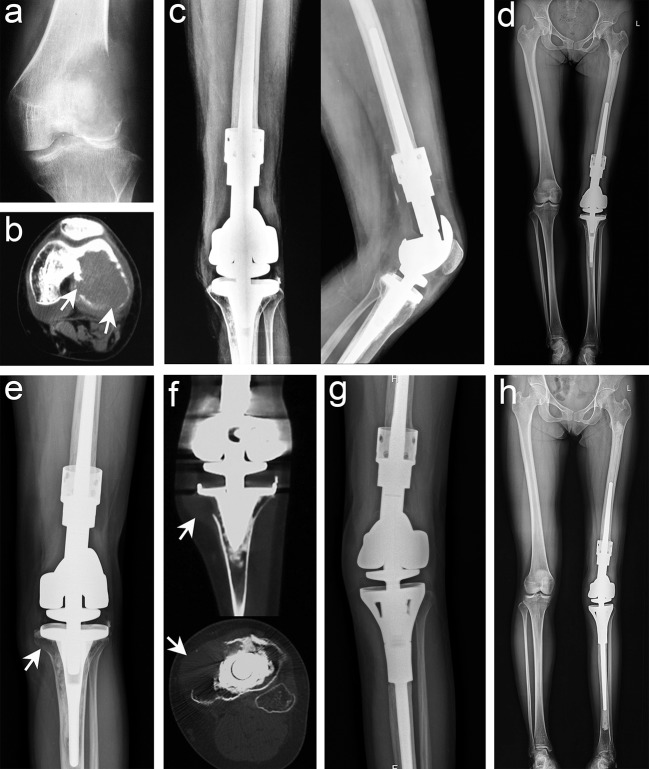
Typical imaging manifestations of patients with recurrence treated with segmental resection. (a) Anteroposterior radiographs of recurrence after curettage in other hospitals. (b) Computed tomography (CT) image showing that the lesion had penetrated the bone cortex and involved the intercondylar fossa and the posterior part of the joint. (c,d) Anteroposterior, lateral, and total length of lower limb radiographs after segmental resection and artificial prosthesis reconstruction. (e) Osteolytic lesions were found in the proximal tibia 15 months after the operation. (f) CT scan confirmed that the lesion invaded the peripheral tissues. (g,h) Anteroposterior and total length on the lower limb radiographs after segmental resection was performed again and artificial prosthesis reconstruction (white arrow points to the lesion).
